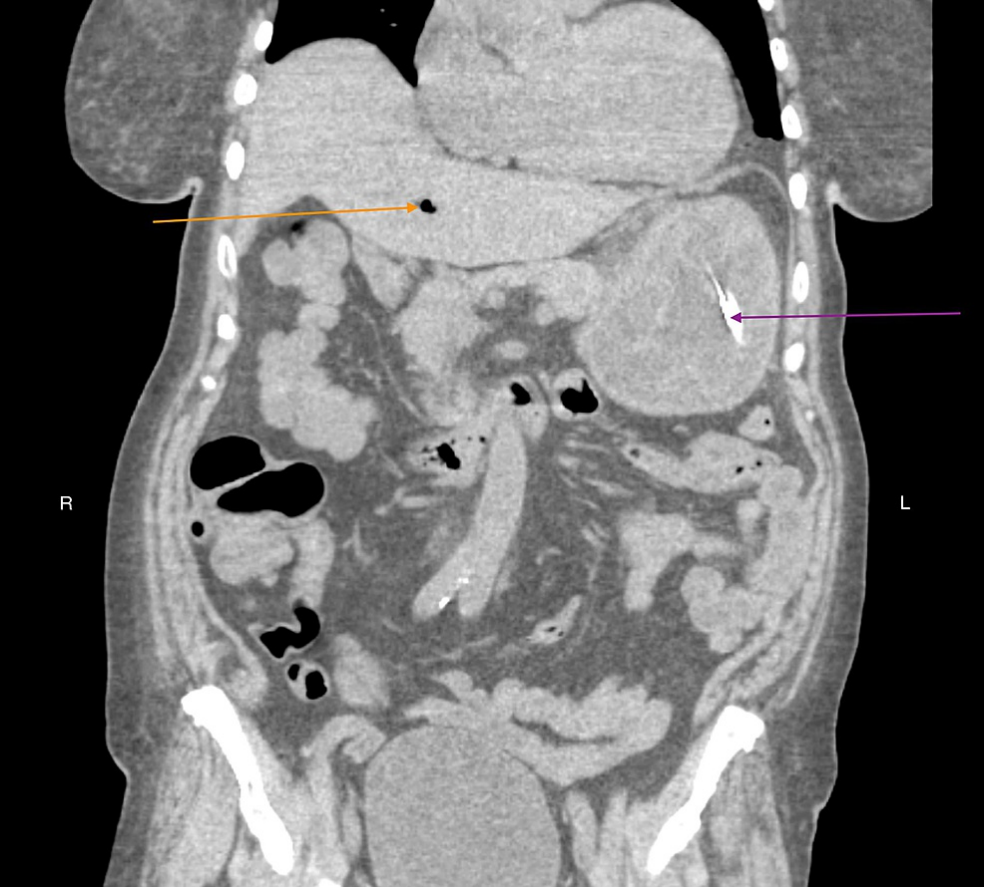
CT scan of abdomen without contrast (coronal view) demonstrating marked improvement in gastric wall edema, nasogastric tube in situ (violet arrow), and minimal gas in main portal vein (orange arrow).
